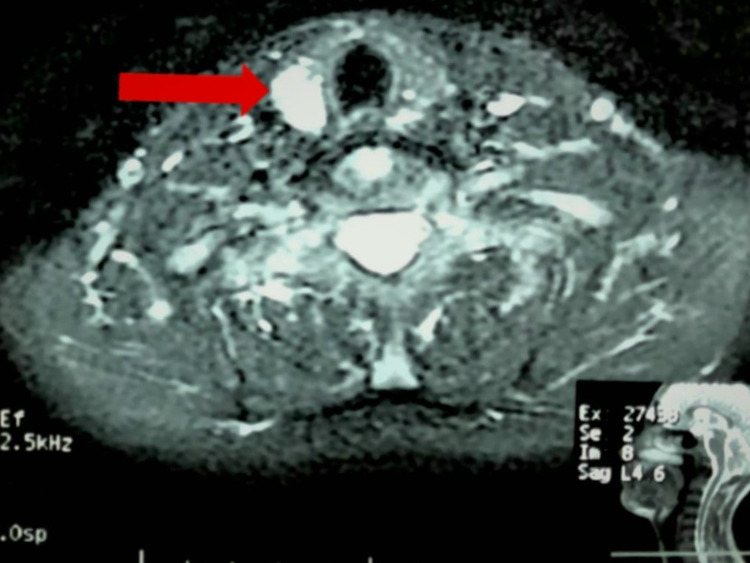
Parathyroid adenoma visualized on MRI contrast of the neck MRI: Magnetic resonance imaging
